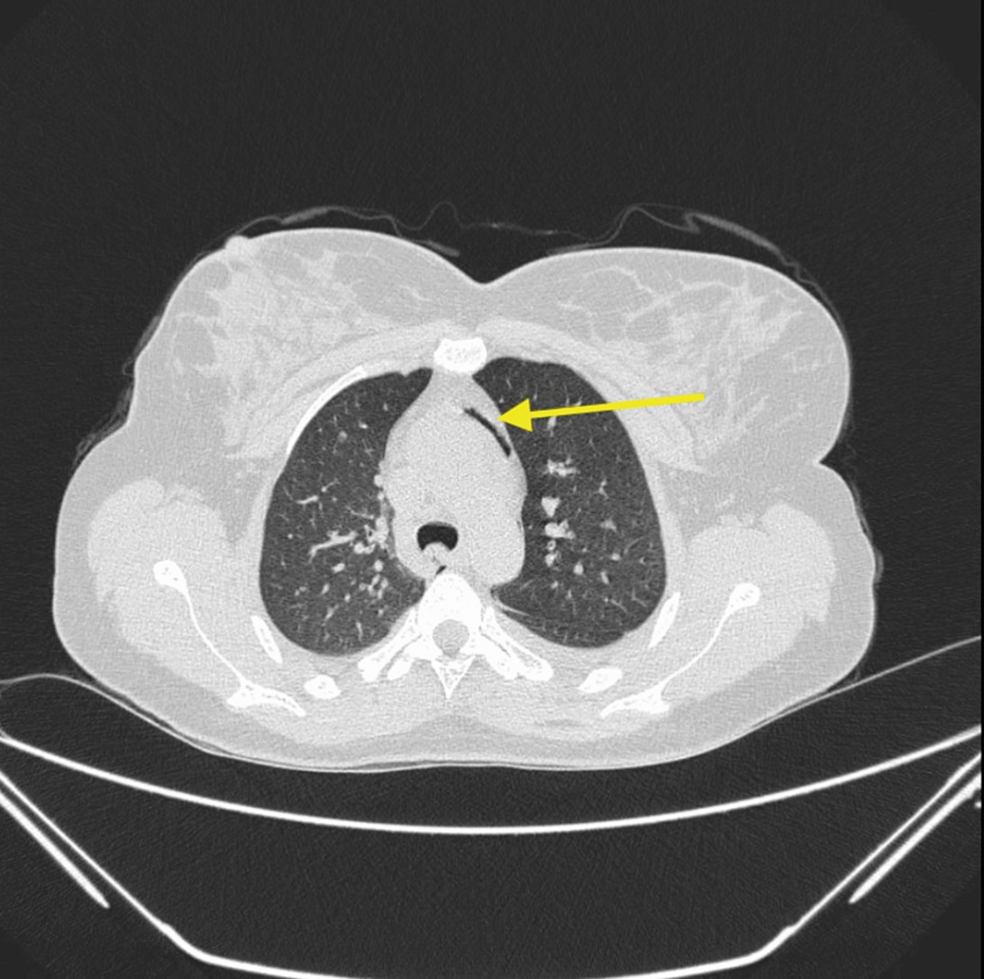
High-resolution computed tomography (HRCT) thorax with pneumomediastinum in the neck (yellow arrow)
